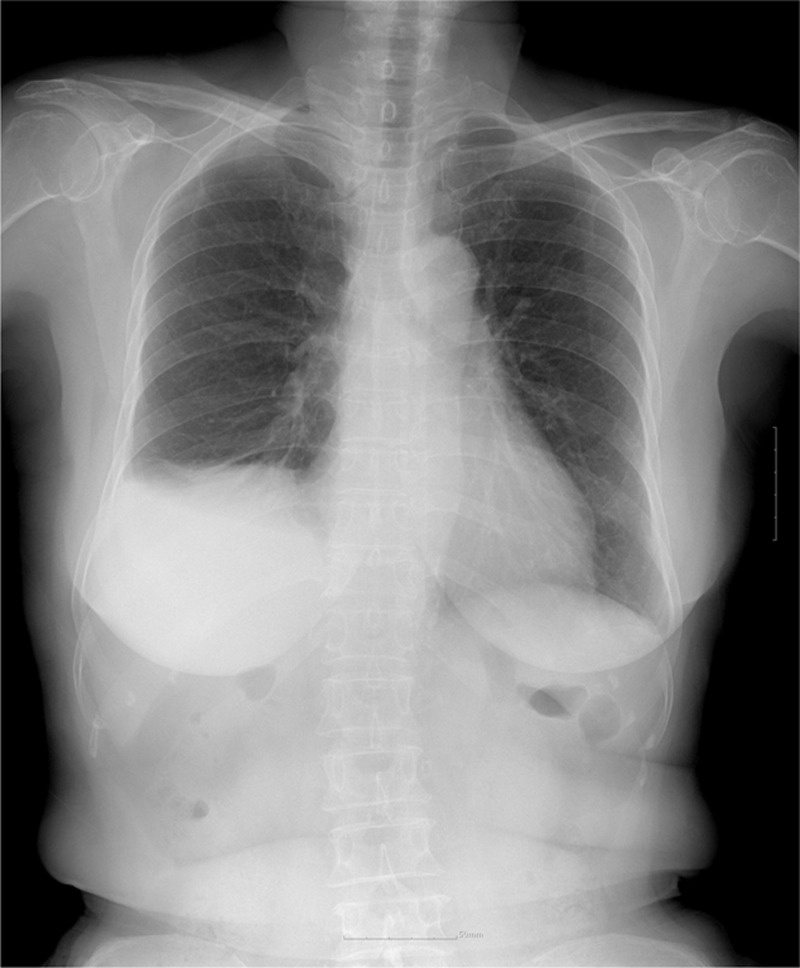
Chest X-ray at 3 months showing an elevation of the right hemidiaphragm associated with basal atelectasis of the right lower lobe and pleural effusion.
